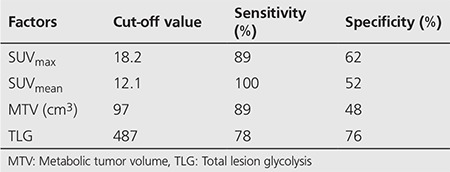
Cut-off value, sensitivity and specificity rates of SUV_max_, SUV_mean_, metabolic tumor volume and total lesion glycolysis in high-grade primary extranodal lymphomas of the gastrointestinal system
